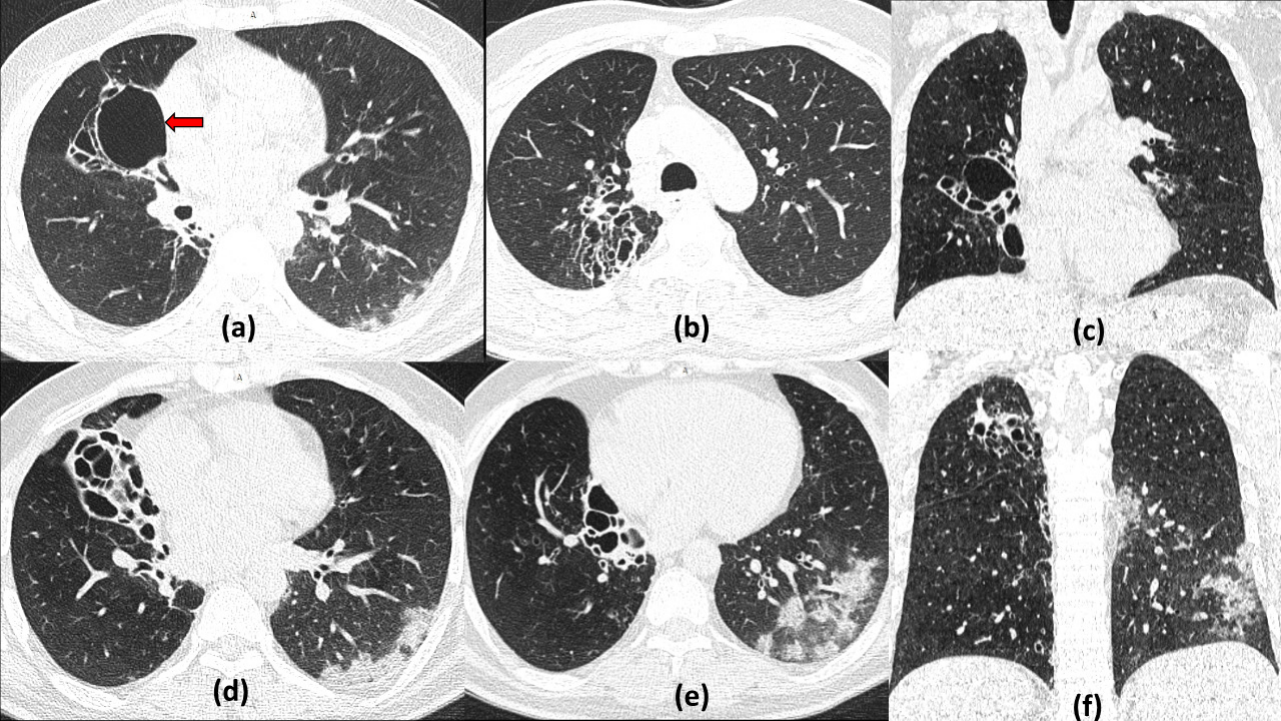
Cystic bronchiectasis with COVID19 infection mimicking cavities: 48-year-old male with history of previous lower respiratory tract infection and now RTPCR positive for COVID19 infection. Axial and coronal chest HRCT sections on Day 18 of illness show a large cystic lesion with thick walls in the right middle lobe mimicking a cavity (arrow in a). However, on contiguous sections (d) it was observed to be communicating with the trachea-bronchial tree suggestive of cystic bronchiectasis, which was also seen involving the posterior segment of right upper lobe. Multiple patchy peripheral subpleural consolidations are seen in the left lower lobe suggestive of COVID19 pneumonitis.
